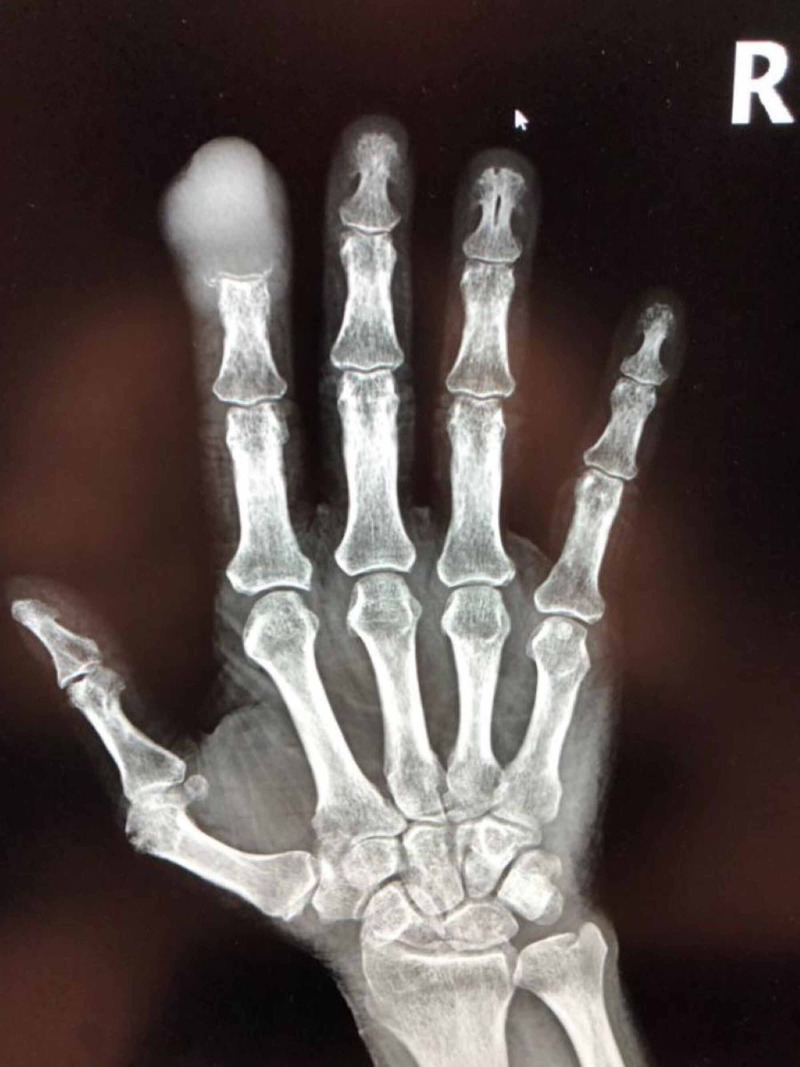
Plain X-Ray of the involved hand taken at admission.
